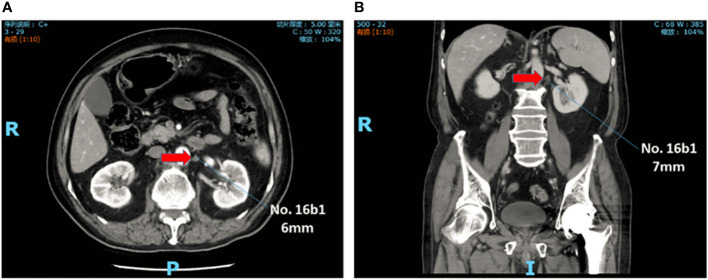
sPAN in abdominal contrast-enhanced computed tomography scan. The lymph node, with diameter less than lcm, was detected at No. 16b1. (A) Transverse plane. (B) Reconstructed image in coronal plane. sPAN, small para-aortic lymph node.
